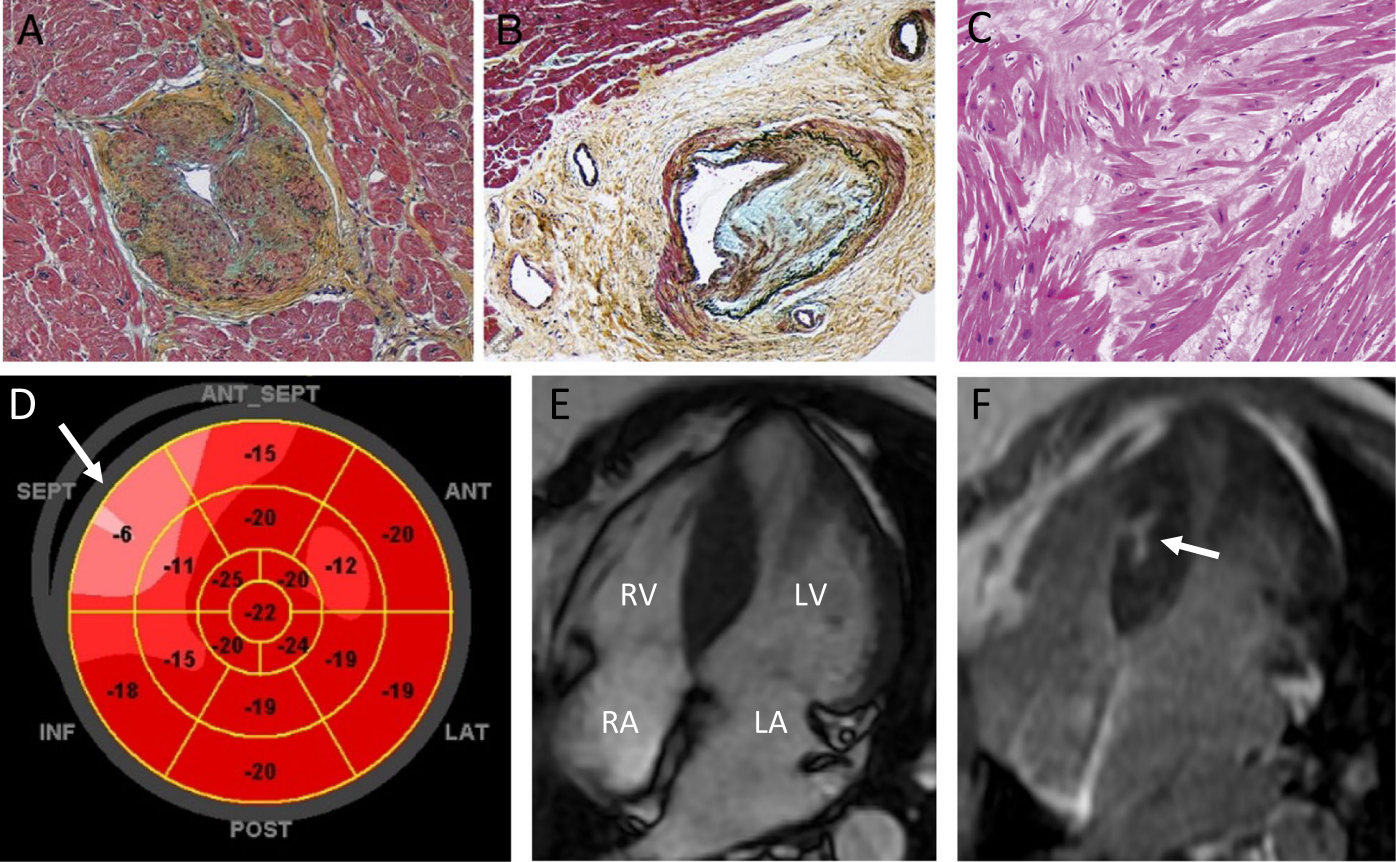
Pathological and imaging findings in hypertrophic cardiomyopathy. The top row shows examples of pathological findings in hypertrophic cardiomyopathy - small intramural coronary arteriole dysplasia, with narrowed lumen (a and b, reproduced with permission from [54]), and interstitial fibrosis with widespread myocyte disarray (c, reproduced with permission from [55]). The bottom row shows imaging findings in a single patient. Speckle tracking echocardiography shows segmental impairment in longitudinal systolic strain, particularly in the base to mid inferior septum (d, arrow). Global longitudinal strain was normal, at − 19%. Cardiac MRI shows septal hypertrophy (e) with late gadolinium enhancement (f, arrow), corresponding to the area of impaired strain. Abbreviations: LA, left atrium; LV, left ventricle; RA, right atrium; RV, right ventricle
